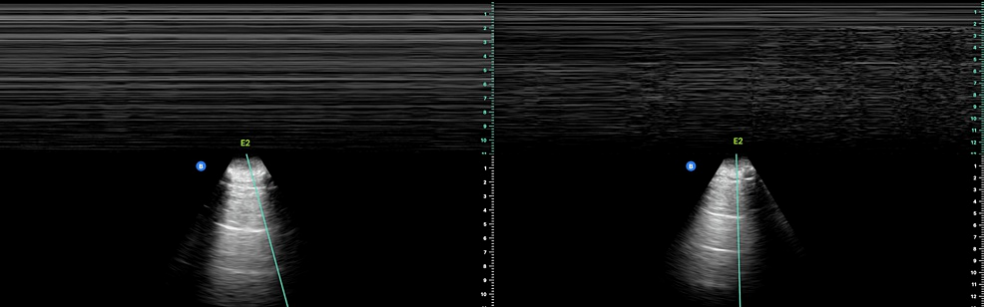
Pneumothorax. These are thoracic M-mode ultrasound images (upper part of the images), accompanied inferiorly by the respective B-mode selected location. On the left, there is only a barcode/stratosphere sign (M-mode evidence of pneumothorax) indicating no lung sliding due to air interposition. On the right, it is possible to see transitions from the seashore sign (M-mode evidence of normal lung sliding) to the barcode/stratosphere sign. This allows the determination of the “lung point sign,” an ultrasound finding that represents the transition between sliding lung and pneumothorax. This sign is highly specific for pneumothorax, showing the point where the visceral pleura begins to separate from the parietal pleural at the margin of the pneumothorax. According to its location, the “lung point” may also indicate the size of the pneumothorax. Patient consent was obtained for the use of anonymous medical data in this paper.
